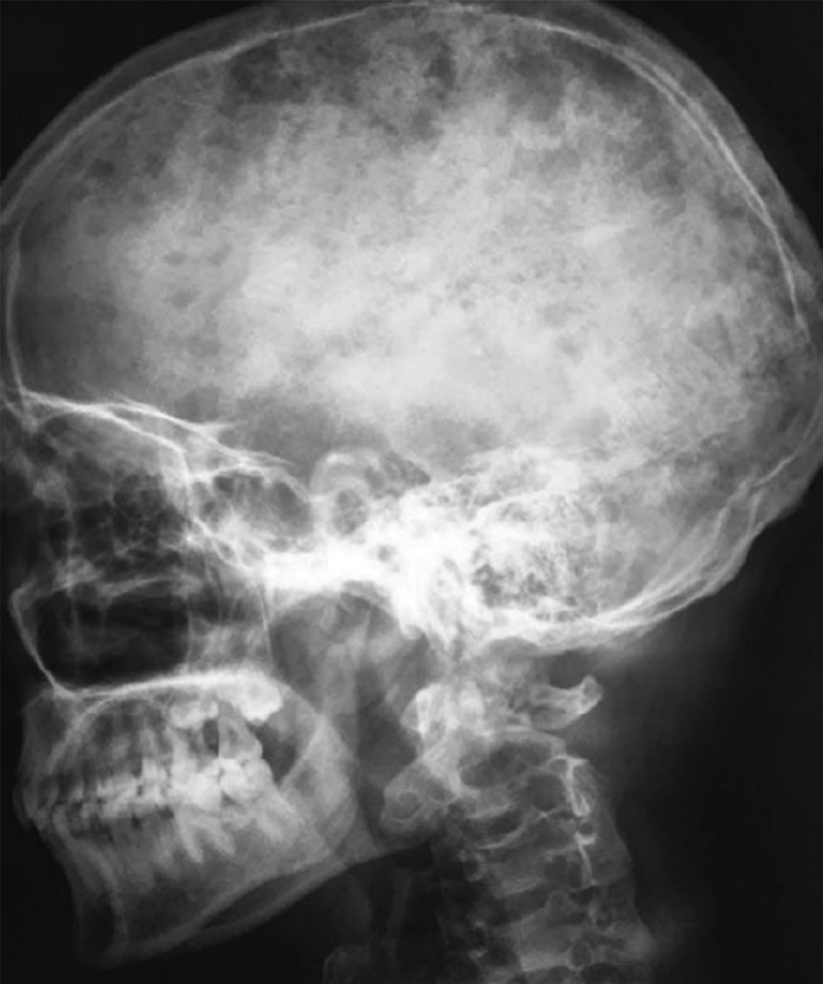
Radiograph of the skull showing multiple osteolytic lesions giving a characteristic “pepper-pot” appearance.
